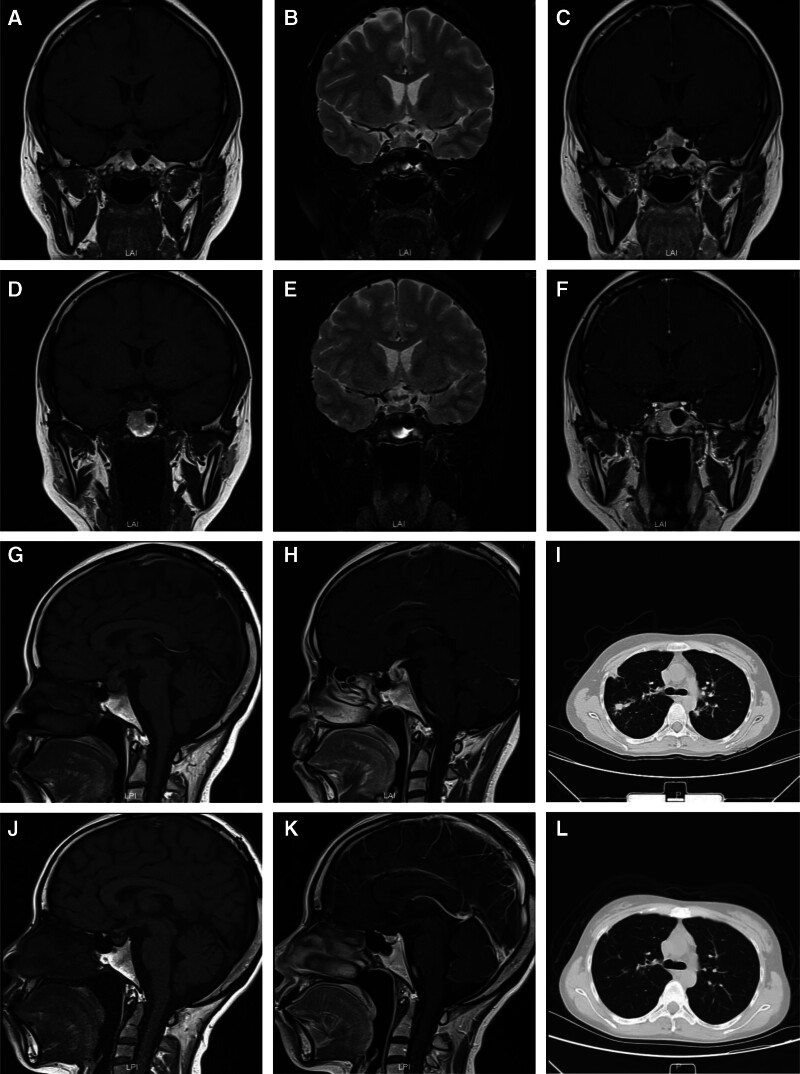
Imaging findings in case 2. Pituitary MRI T1-weighted (A, G): enlargement and thickening of the pituitary gland (11 mm × 10 mm) and infundibulum with abnormal signal intensity; T2-weighted (B): shows moderate signal intensity of the pituitary gland; enhanced pituitary MRI (C, H): heterogeneous contrast enhancement within the pituitary; chest CT scan (I): reveals multiple mass-like shadows in the lungs; follow-up pituitary MRI (D–F, J, K): showed a smaller pituitary gland (10 mm × 6 mm) compared to previous scans; follow-up chest CT scan (L): demonstrates resolution of the masses. MRI = magnetic resonance imaging, CT = computerized tomography.
